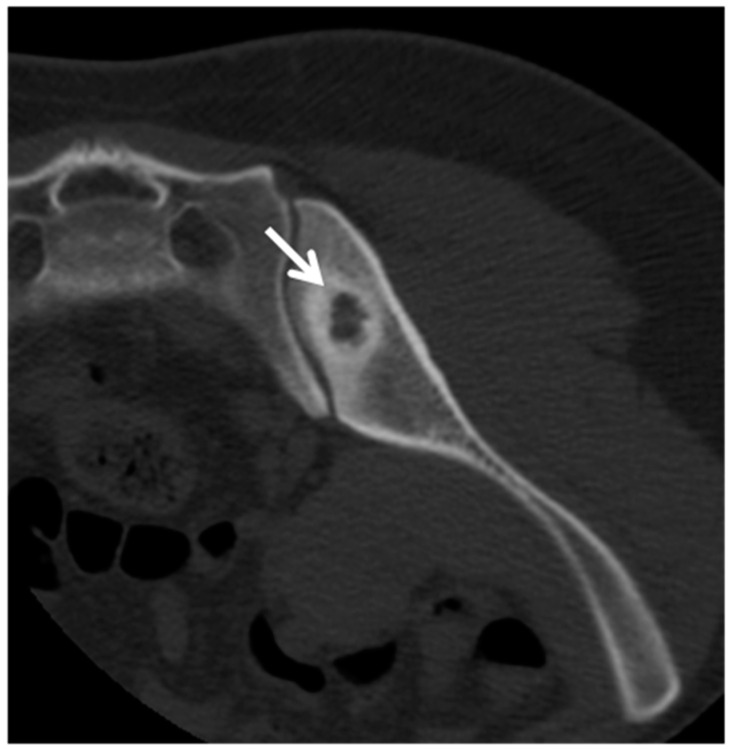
Brodie’s abscess in a 30-year-old man. Computed tomography of the pelvis showed a small (1.5 cm) radiolucent lesion with thick and irregular sclerotic margins (arrow).
